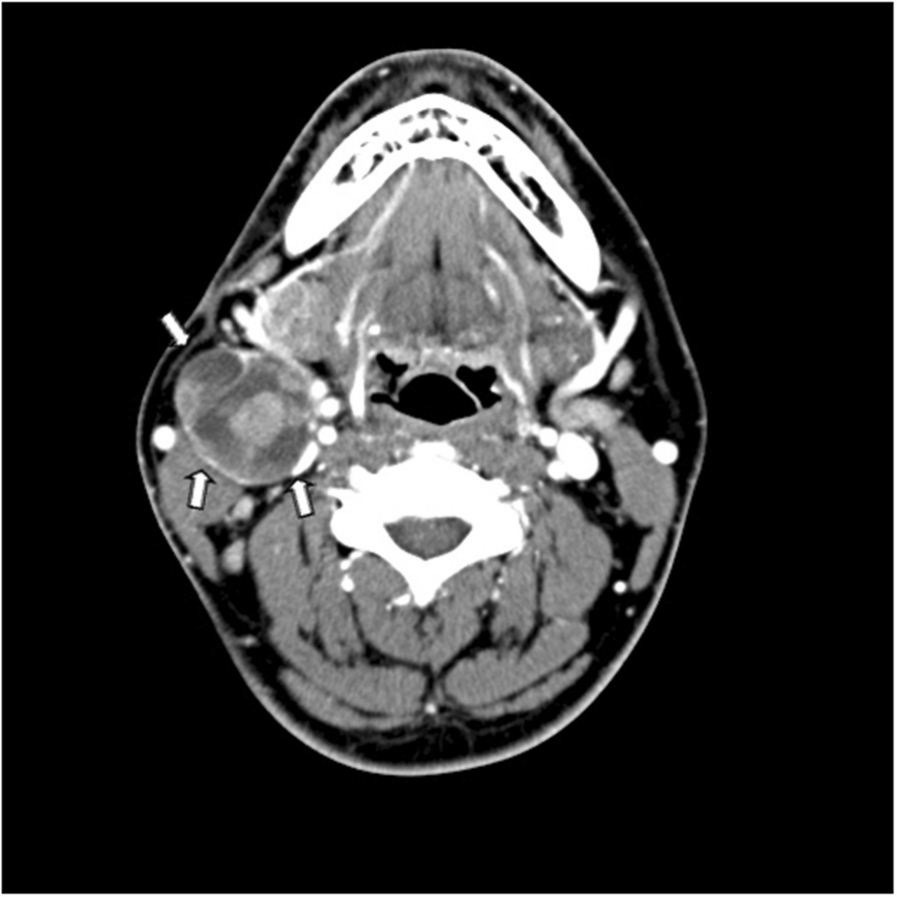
Computed tomography (CT) findings. CT with enhancement showing heterogeneously enhancing mass at right level II (arrows, tumor margin).
